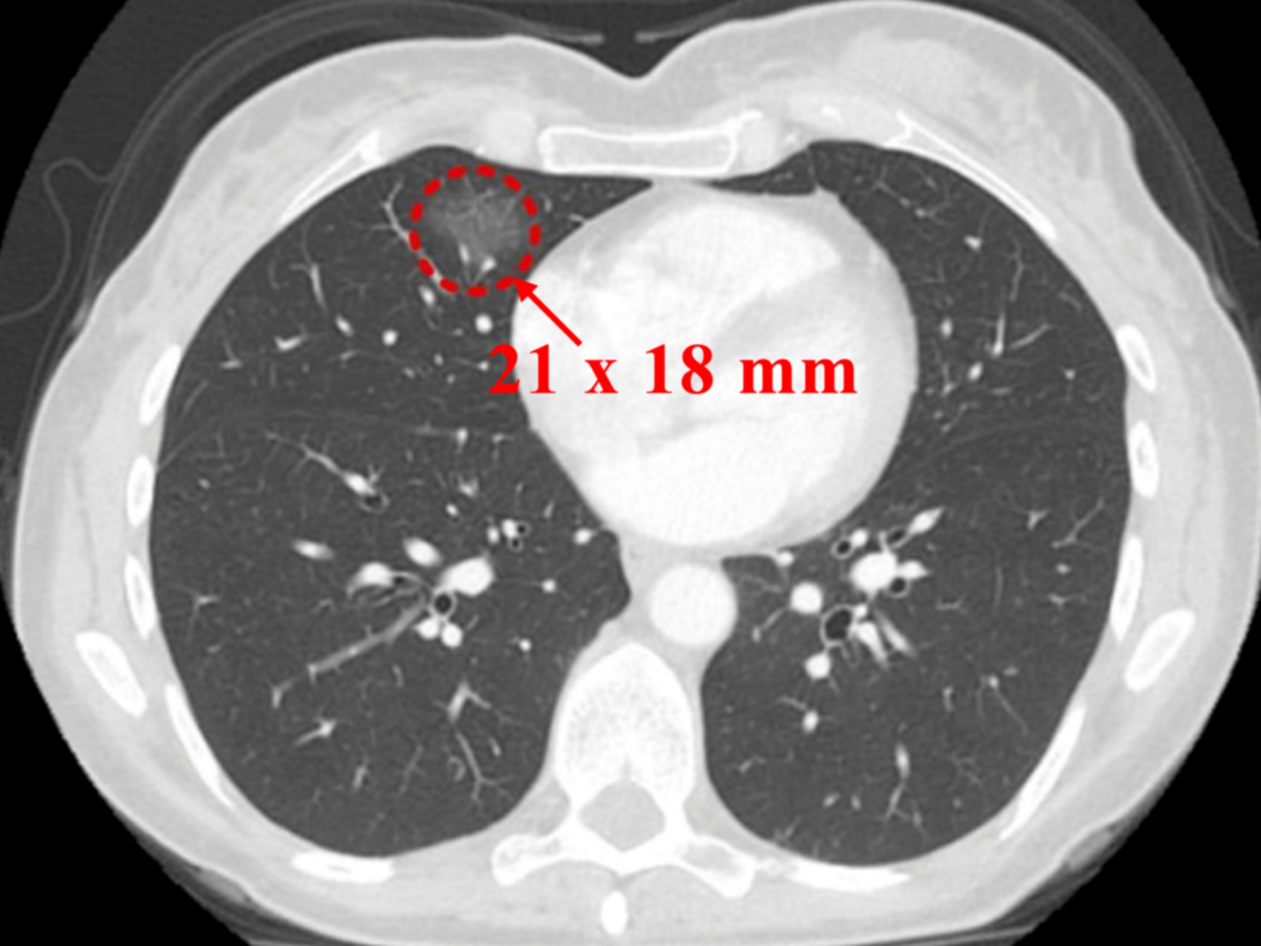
Contrast-enhanced computed tomography (CECT) of the chest (a month following the previous CT)
